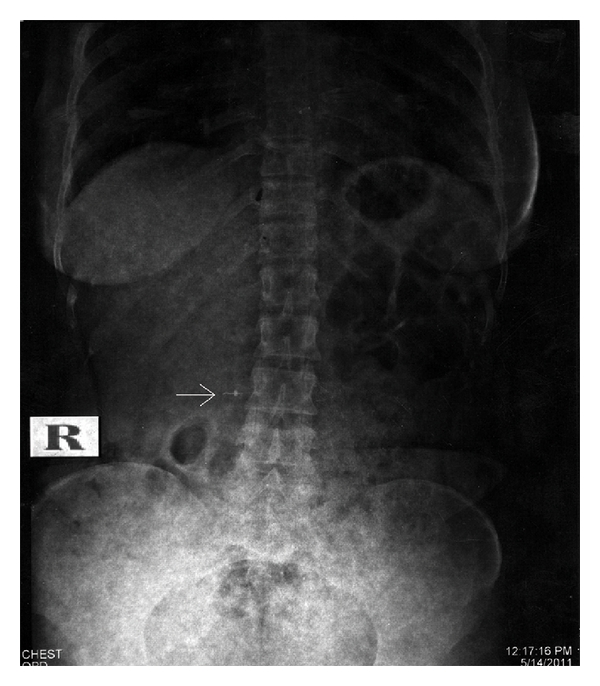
Abdomen radiograph after half an hour, showing instrument in lumbosaccaral region of intestine.
